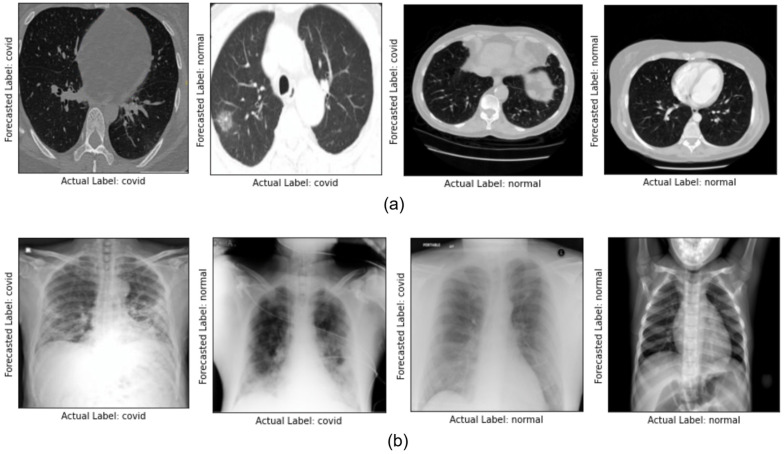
Prediction result of proposed SCovNet model (a) CT scan dataset and (b) CXR Images dataset.
